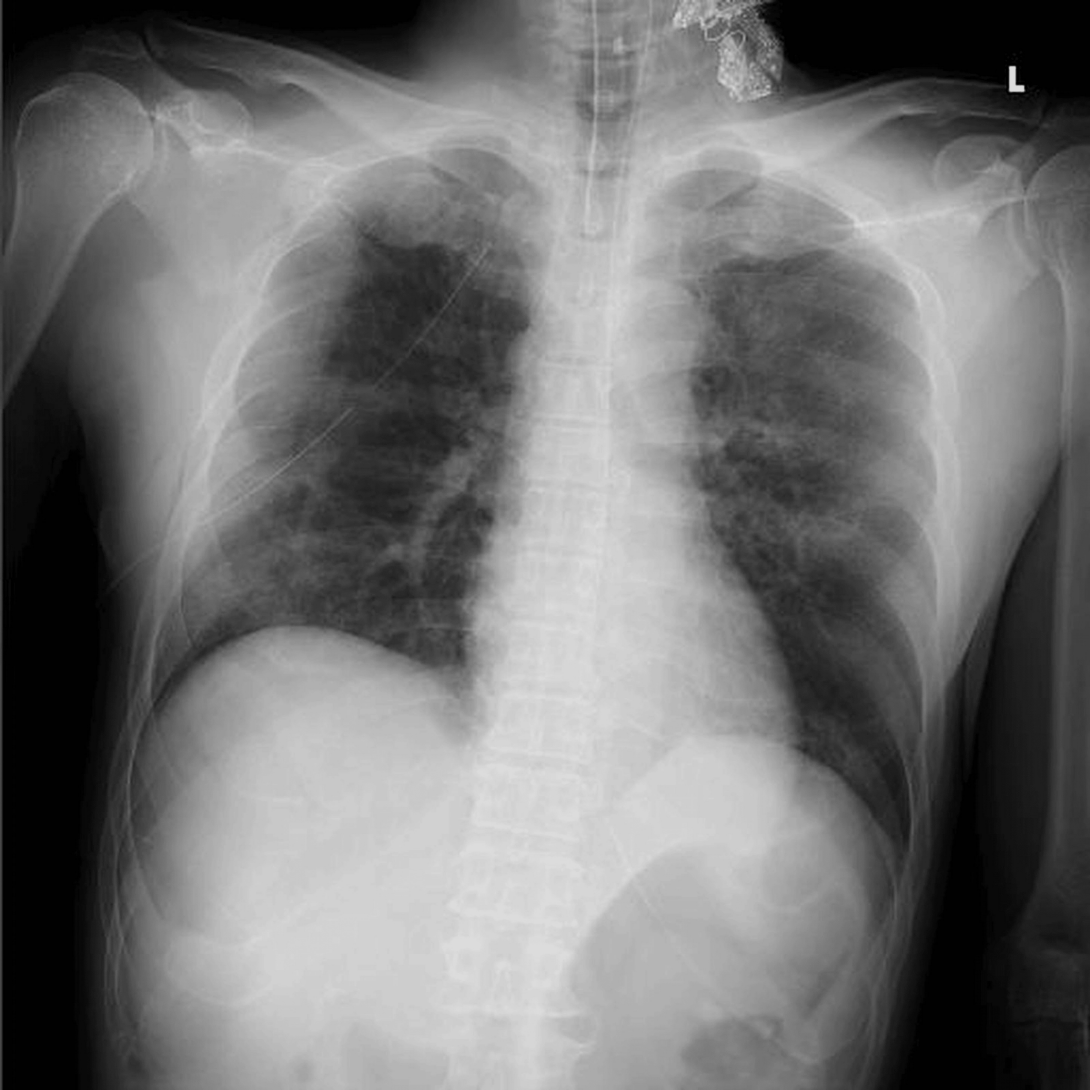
Chest X-ray image on admission The patient was intubated, and a chest drain was placed in the right pleural cavity
